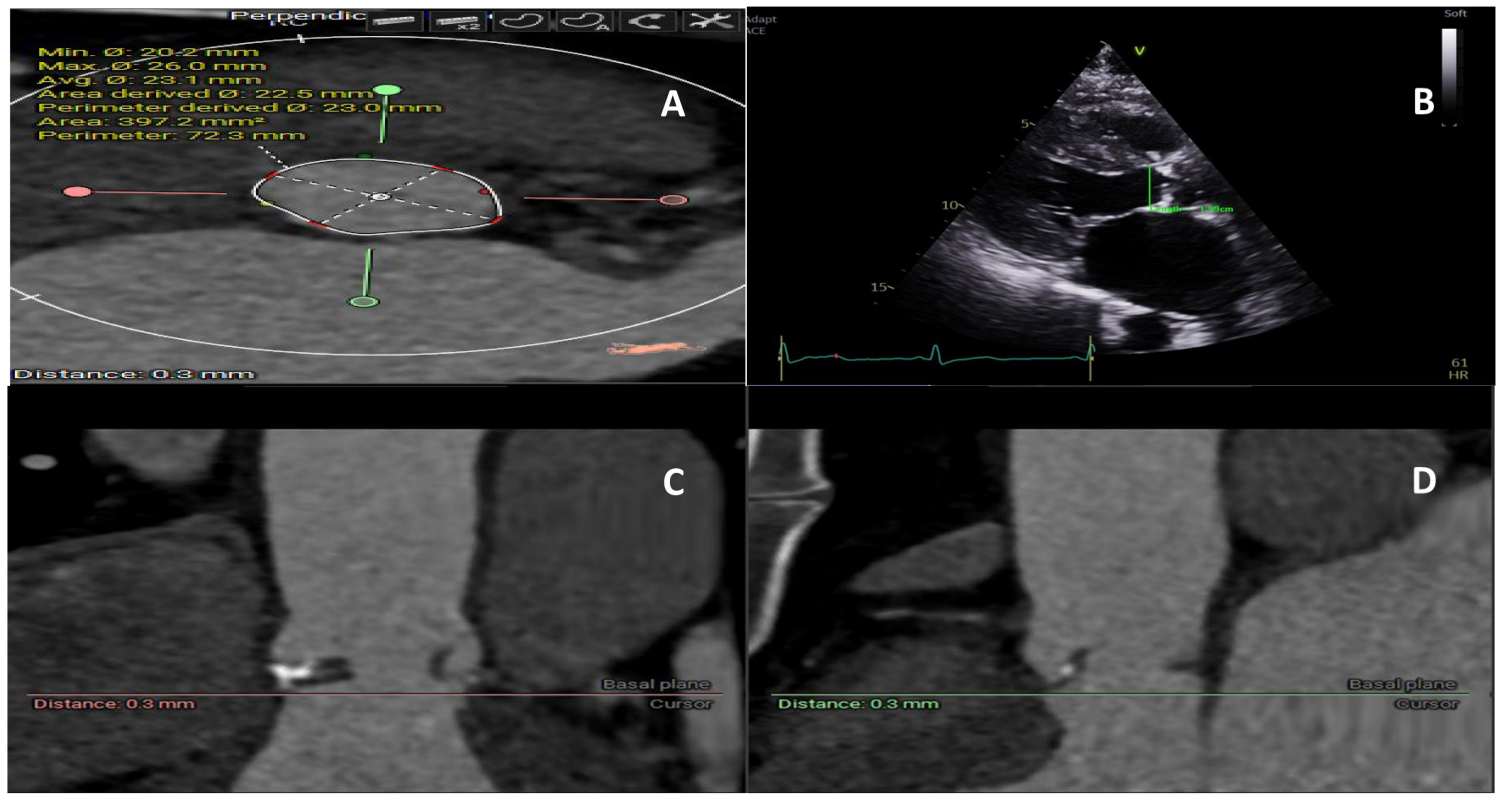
Aortic annulus measurement using computed tomography and echocardiogram. (A) Annulus size measurement on computed tomography providing area and perimeter. (B) Echocardiogram 2D measurement of the one axis of the annulus, (C) Computed tomography section provides a three-dimensional view of the annulus and its relationship to the surrounding structure. (D) Computed tomography section provides a three-dimensional view of the annulus and its relationship to the surrounding structure.
